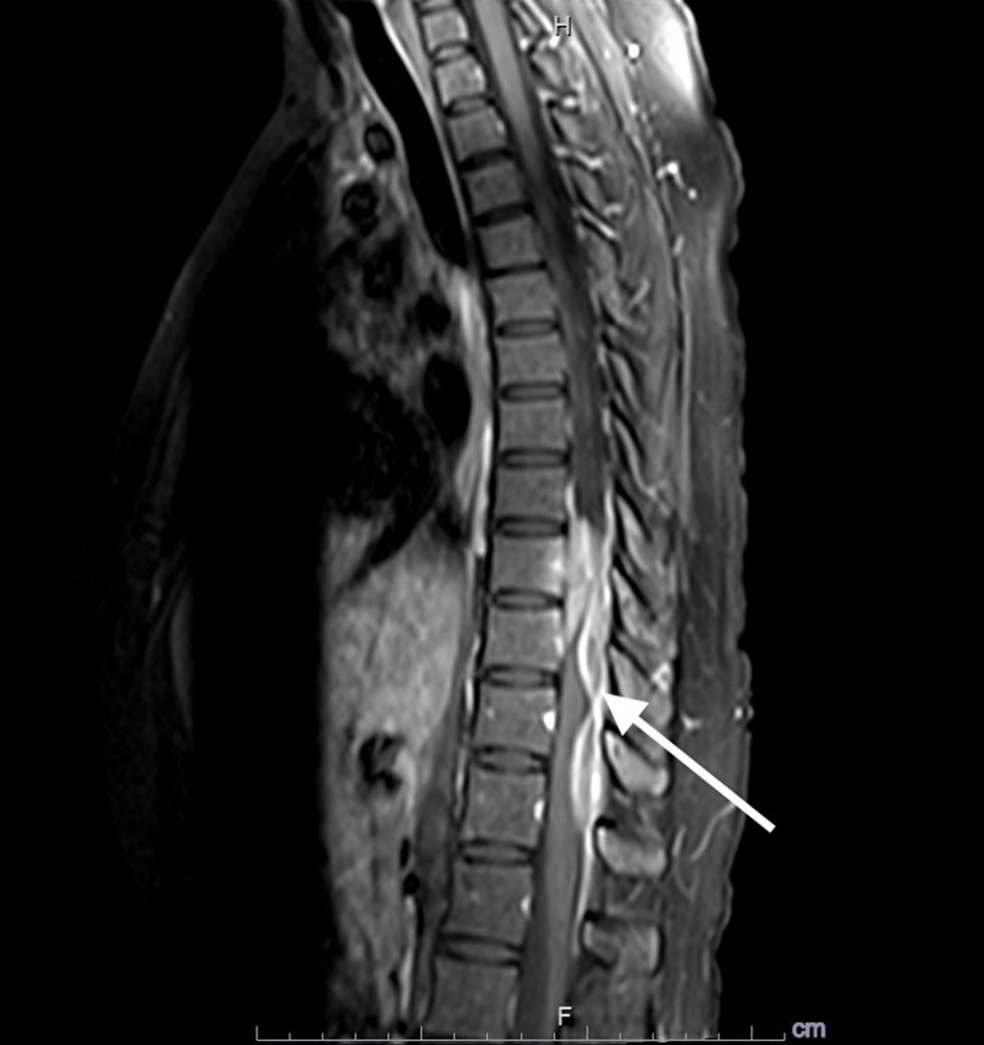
Initial magnetic resonance imaging (MRI) of the thoracic spine eight years before the current presentation. MRI of the thoracic spine showing two extramural enhancing lesions with associated spinal canal stenosis and spinal cord compression (arrow).
